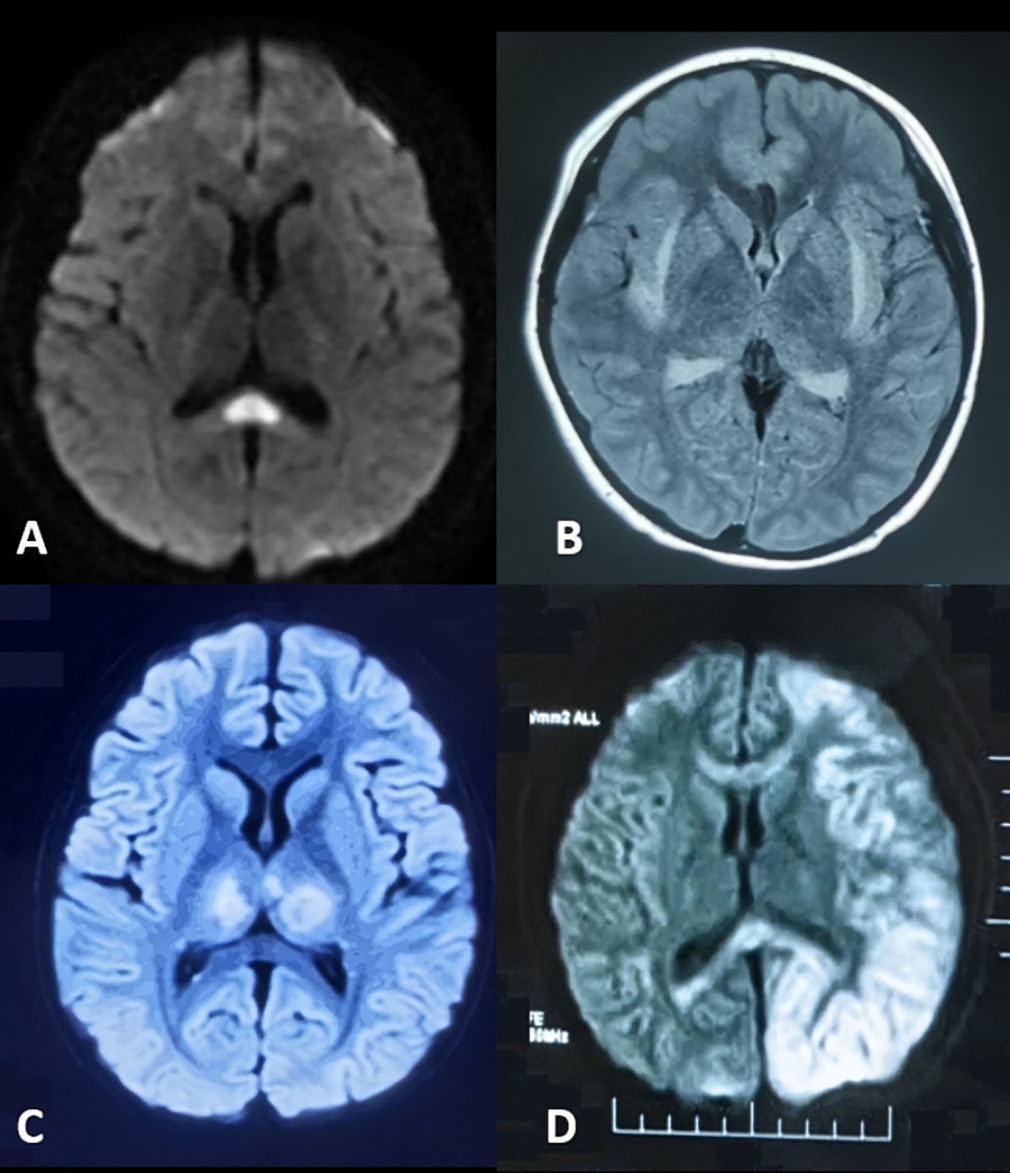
Neuroimaging of parainfectious encephalopathies. (A) Mild Encephalopathy with Reversible Splenial Lesion: splenium of the corpus callosum hyperintensity on diffusion-weighted imaging (DWI). (B) Febrile Infection-Related Epilepsy Syndrome with Claustrum Lesion: bilateral claustrum hyperintensity on T2-weighted and fluid-attenuated inversion recovery (FLAIR) imaging. (C) Acute Necrotizing Encephalopathy: bilateral thalamic hyperintensity on T2/FLAIR imaging. (D) Hemiconvulsion-Hemiplegia Syndrome: left cerebral cortex and subcortical hyperintensity on DWI with corpus callosum involvement and associated edema.
